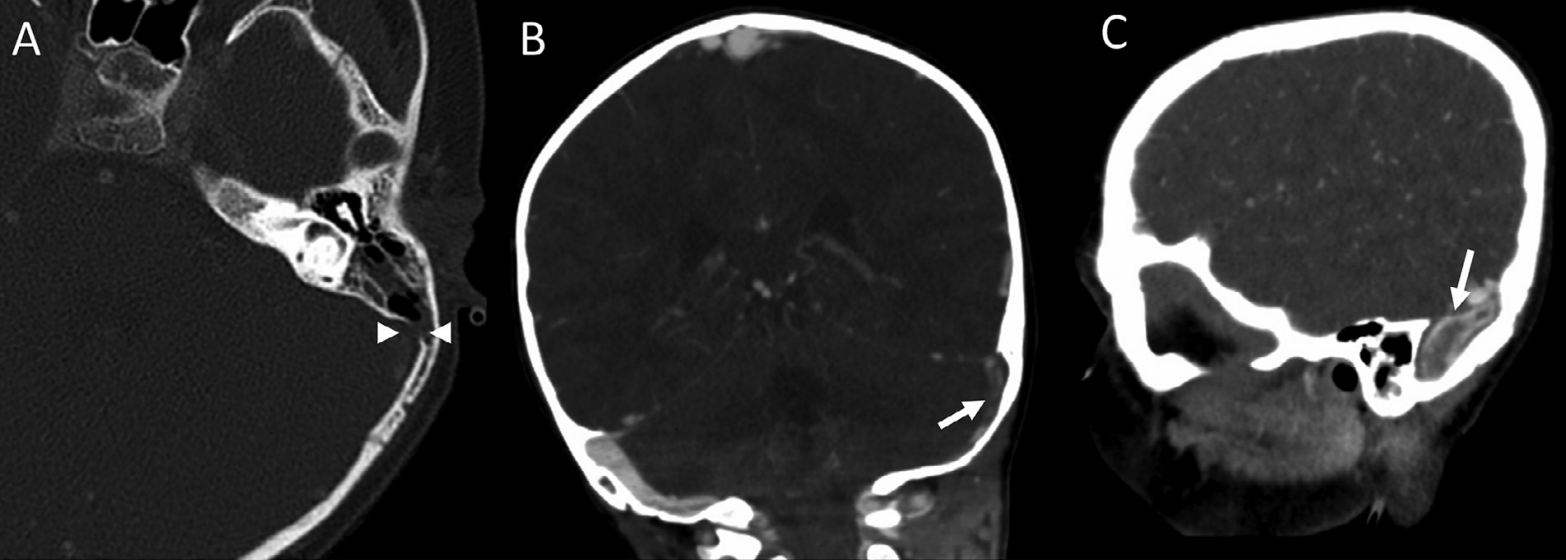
Computed tomographic venography (CTV). Axial (A) image demonstrates marked thinning of the left sigmoid sinus bony plate (arrowheads) caused by chronic mastoiditis. Coronal (B) and sagittal (C) images demonstrate iodinated contrast flowing around the thrombus, consistent with an incompletely occluded sigmoid sinus (arrows).
